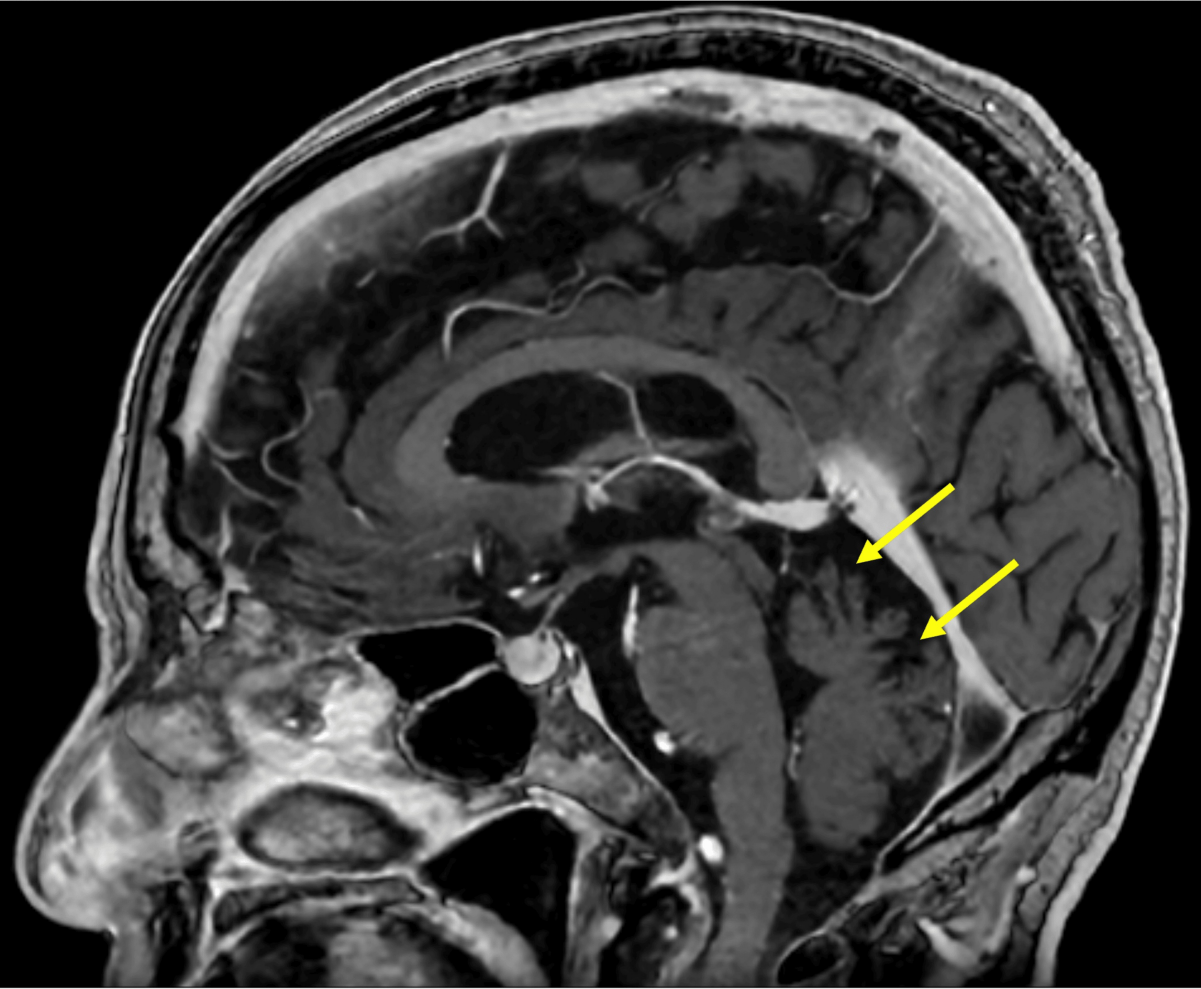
Magnetic resonance imaging of the head Gadolinium-enhanced magnetic resonance imaging that was performed one month prior to the surgery clearly demonstrates atrophy and foliation of the cerebellar cortex (arrows).
